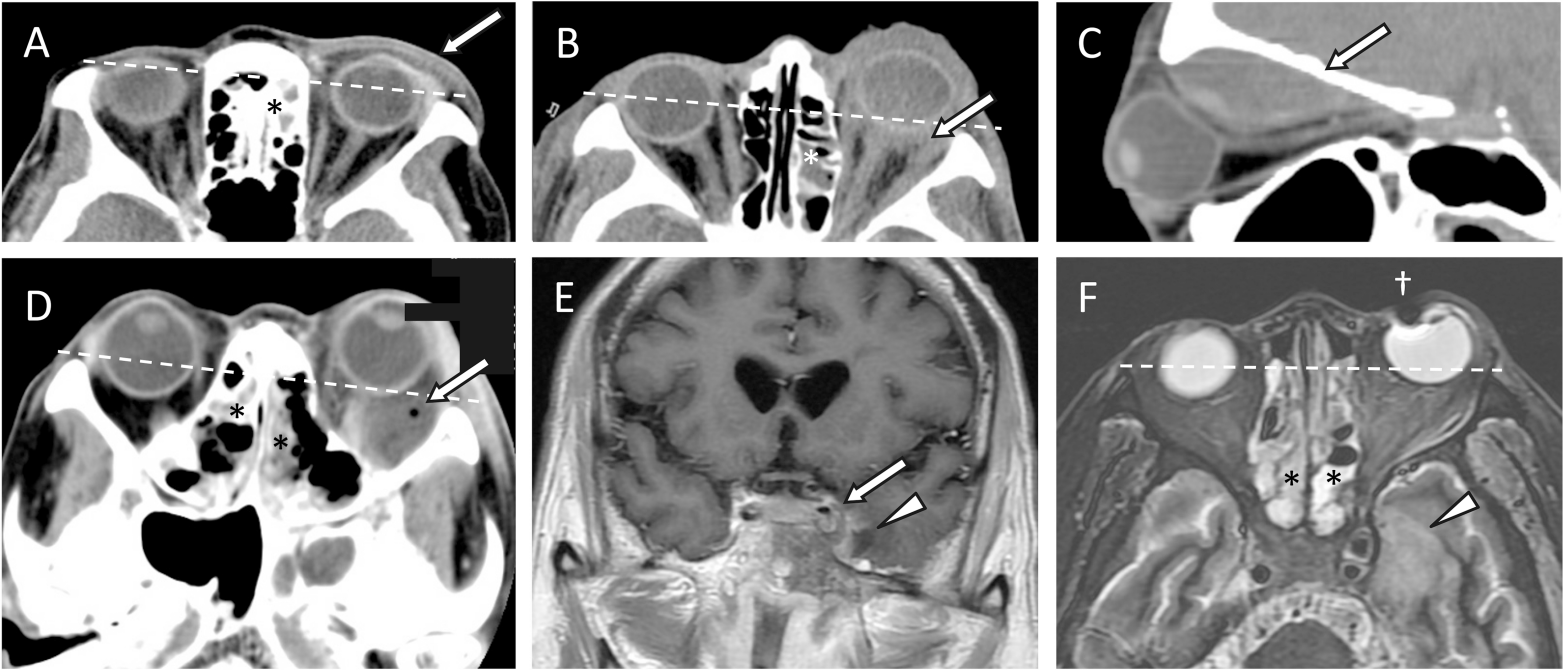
Characteristic findings of each stage of sinusitis-related orbital complications determined via computed tomography (CT) or magnetic resonance imaging (MRI). (A) Stage I, preseptal cellulitis (arrow) on an axial CT image. (B) Stage II, orbital cellulitis (arrow) on an axial CT image. (C) Stage III, subperiosteal abscess (arrow) on a sagittal CT image. (D) Stage IV, orbital abscess (arrow) with a tiny air bubble on an axial CT image. (E and F) Stage V, cavernous sinus thrombosis (arrow) on a coronal contrast-enhanced T1-weighted MRI, and focal cerebritis (arrowheads) on a coronal contrast-enhanced T1-weighted MRI and an axial T2-weighted MRI. * Ethmoidal sinusitis. † Artifact due to metal material. Dashed lines: Proptosis in one eye compared with the fellow eye.
